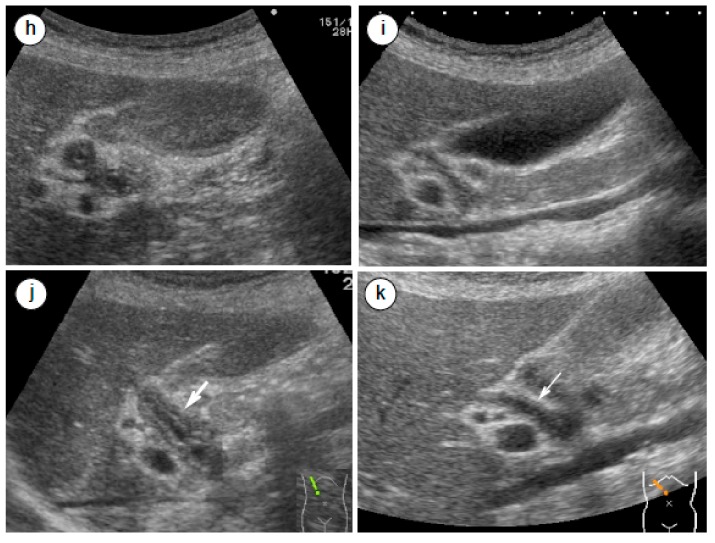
A case of Mikulicz disease with autoimmune pancreatitis (AIP) accompanied by multiple immunoglobulin G4 (IgG4)-related systemic lesions in a 70 year-old female patient. (a) Visualization by 18F-fluorodeoxyglucose-positron emission tomography (FDG-PET) of systemic inflammatory lesions with abnormal FDG uptake (the arrow heads indicate from the top to the bottom: dacryoadenitis, sialadenitis, mediastinum lymphadenopathy, hepatic pseudotumor, autoimmune pancreatitis, and choledocho-chlecystitis. (b) Disappearance of the lesions 3 months after steroid initiation. (c) Computed tomography before steroid treatment demonstrated an enlarged pancreas with a capsule-like rim (thin arrow) and markedly thickened hilar bile duct (thick arrow). (d) Computed tomography 1 year after steroid initiation revealed pancreatic parenchymal shrinkage and improved bile duct thickness. (e) Abdominal ultrasonography before (f,h,j) and 2 months after (g,i,k) steroid therapy showed a dramatic improvement in the size of the pancreas, as well as wall thicknesses of the gallbladder up to the hilar bile duct (white arrows).
